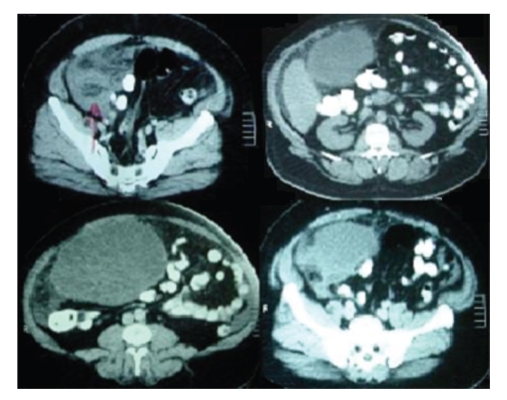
Abdominal CT scan showing a huge heterogeneous cystic mass occupying the entire right side of the abdomen and extending down to the pelvis.
